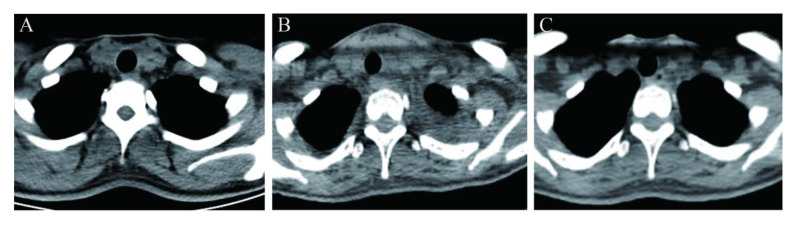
Neck CT images showing the remaining left thyroid lobe before ^131^I administration (A), and 3 days (B) and 9 days (C) post ^131^I administration.
